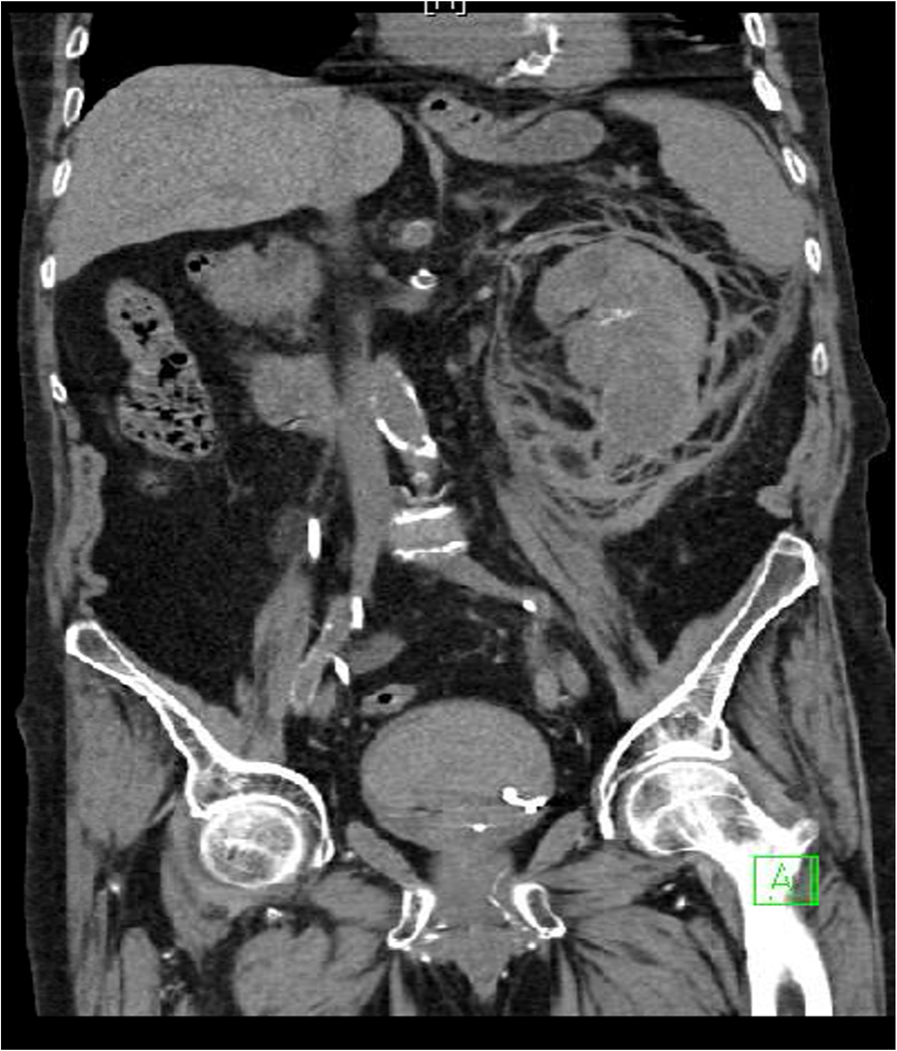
Coronal view of the non-contrast CT scan showing severe left-sided perinephric and retroperitoneal hematoma. It also demonstrates the intra-vesical part of the dislodged double coil stent. Minimal stone fragments are left over
